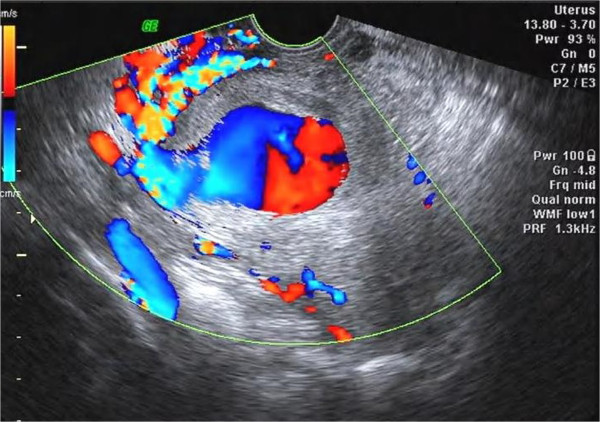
Doppler ultrasonography of the pseudoaneurysm. A swirl of colors, which represents the opening of the pseudoaneurysm and its supplying artery, is visible in this color Doppler ultrasonography image.
